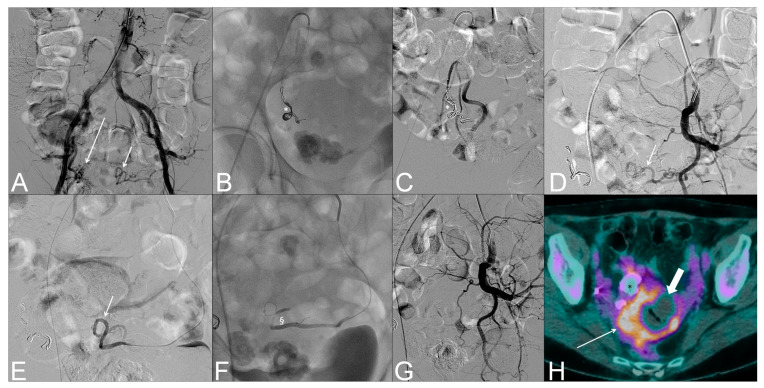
Digital Subtraction Angiography (A–G) and subsequent 18-FDG-PET/CT (H) of a 70-year-old woman with recently diagnosed endometrial cancer, COVID-19 infected, presenting at our institution’s Emergency Department with severe metrorrhagia and anemization. (A) Aortography showing pathological uterine angiogenesis (long arrow) fed by the right uterine artery and the left hypertrophic uterine artery (short arrow). (B) Coils (*), gelfoam, and microsphere embolization of the right uterine artery. (C) Right uterine angiography demonstrating exclusion of the pathological uterine angiogenesis; radiopaque coils (*) can be seen. (D,E) Left hypogastric artery (D) and super-selective uterine artery (E) angiographies demonstrating the left hypertrophic uterine artery after coils embolization of the right uterine artery. (F) SquidPeri 34 (§) embolization of the left uterine artery. (G) Left uterine angiography demonstrating exclusion of the pathological uterine angiogenesis H. Post embolization 18-FDG-PET/CT showing inhomogeneous increased uptake (thin arrow) at the level of the known uterine malignancy with a central non-hypermetabolic region with air bubbles within (thick arrow), consistent with central necrosis and residual disease.
